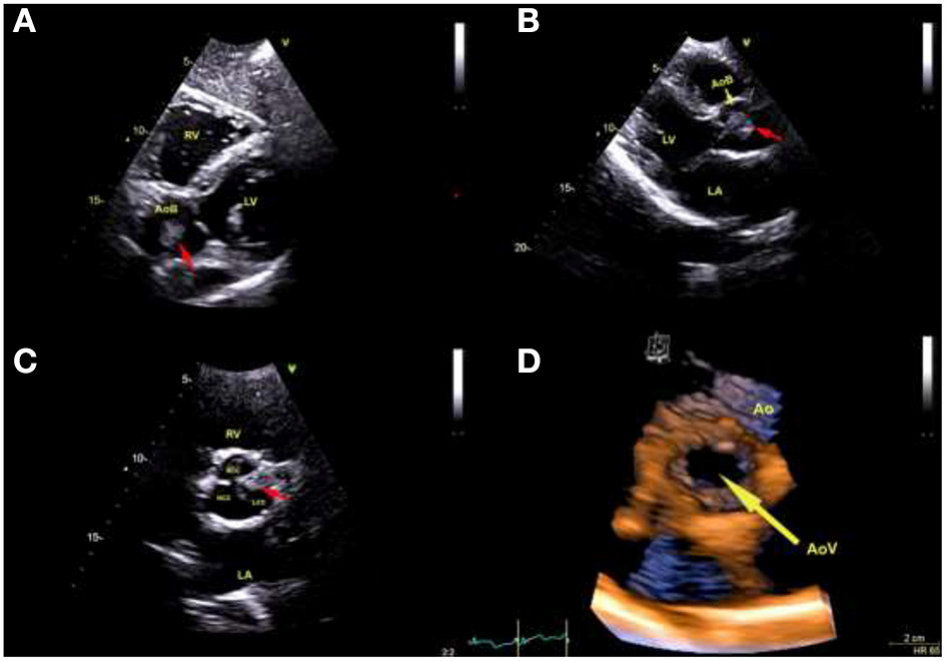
Transthoracic echocardiography: (A) Subxyphoid view shows pedunculated mass (red arrow) localization in Valsalva sinus. (B) Long-axis view measuring tumor size 1.9 × 1.5 cm (red arrow). (C) Short-axis view at aortic valve level demonstrates tumor (red arrow) originating immediately after the separation of the left and right coronary cusp. (RV) Right ventricle. (LV) Left ventricle. (AoB) Aortic bulb. (LA) Left atrium. (RCC) Right coronary cusp. (NCC) Noncoronary cusp. (LCC) Left coronary cusp. (D) Transthoracic three-dimensional echocardiography: view of the aortic valve (yellow arrow) from the aortic side, which shows the aortic cusps' normal appearance. (AoV) Aortic valve. (Ao) Aorta.
